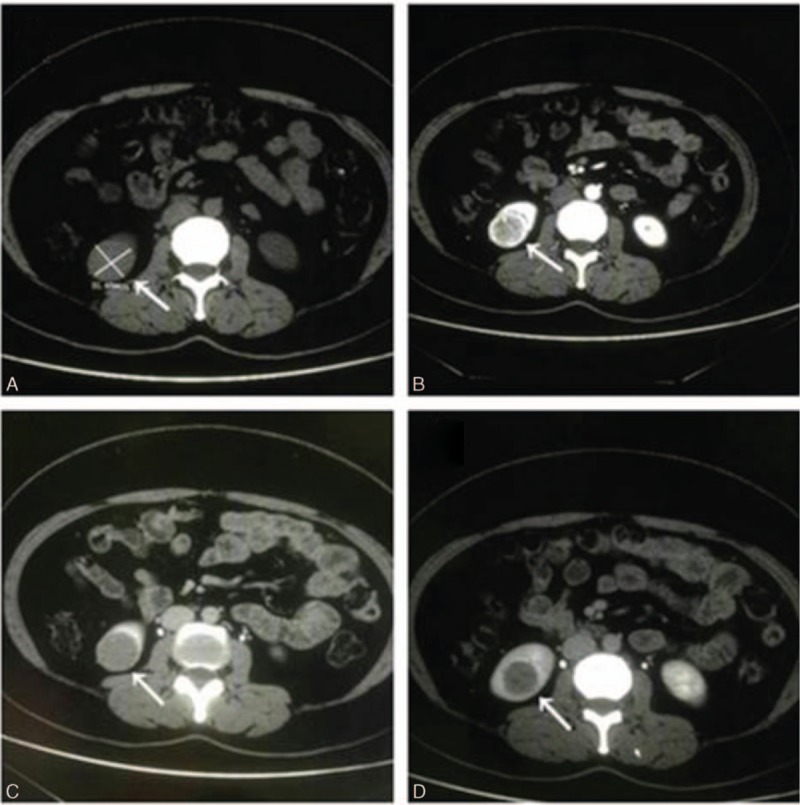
(A) Plain CT scan revealed a 4 × 3 × 3 cm well-demarcated mass on the lower pole of right kidney (white arrow). The tumor was enhanced nonuniformly during arterial phase (B), whereas no enhancement was shown during venous phase (C) and renal parenchymal phase (D). CT = computed tomography.
